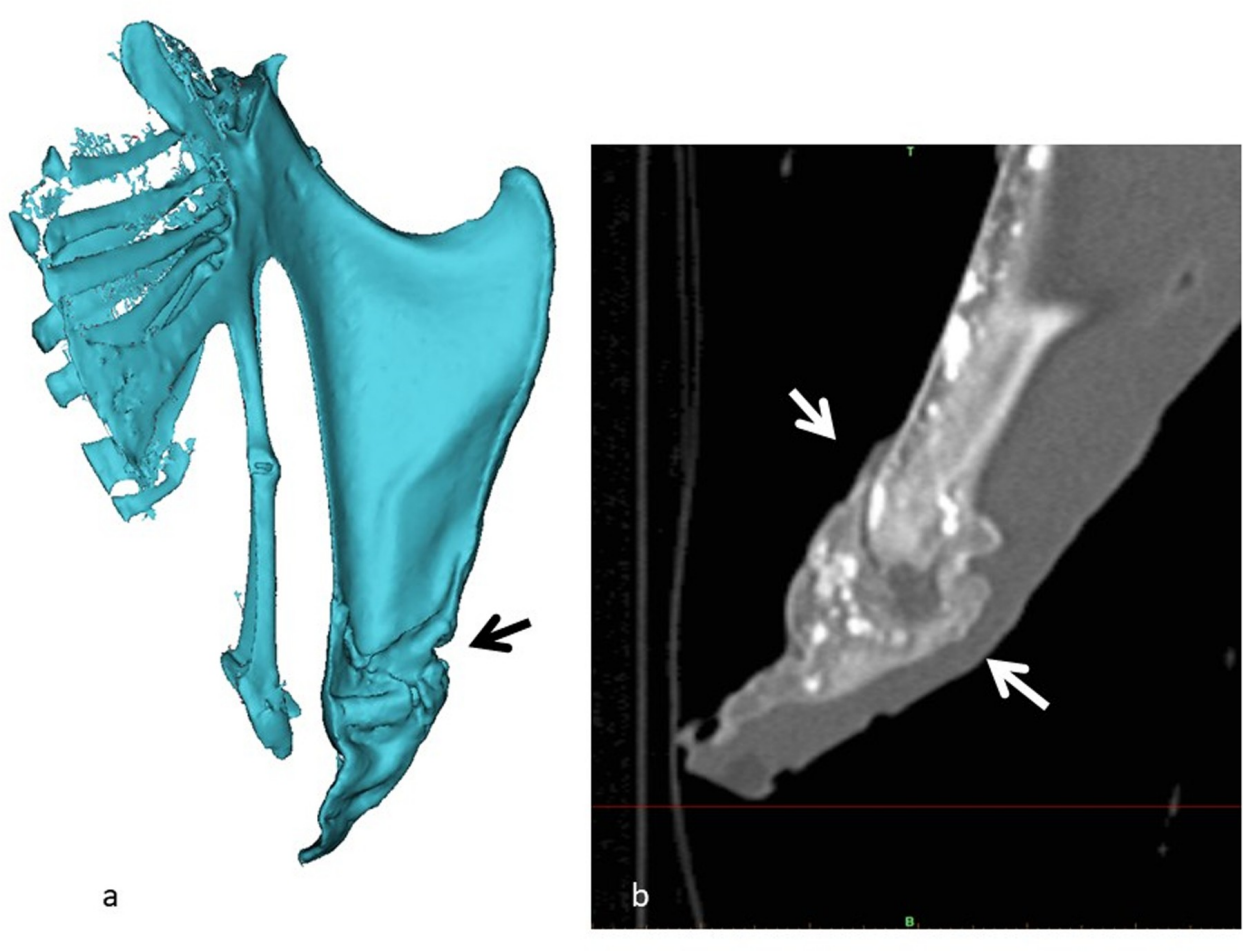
3D model (a) and CT image (b) of a 75+-week hen showing multiple fractures and the so-called pseudoarticulation (arrows).
